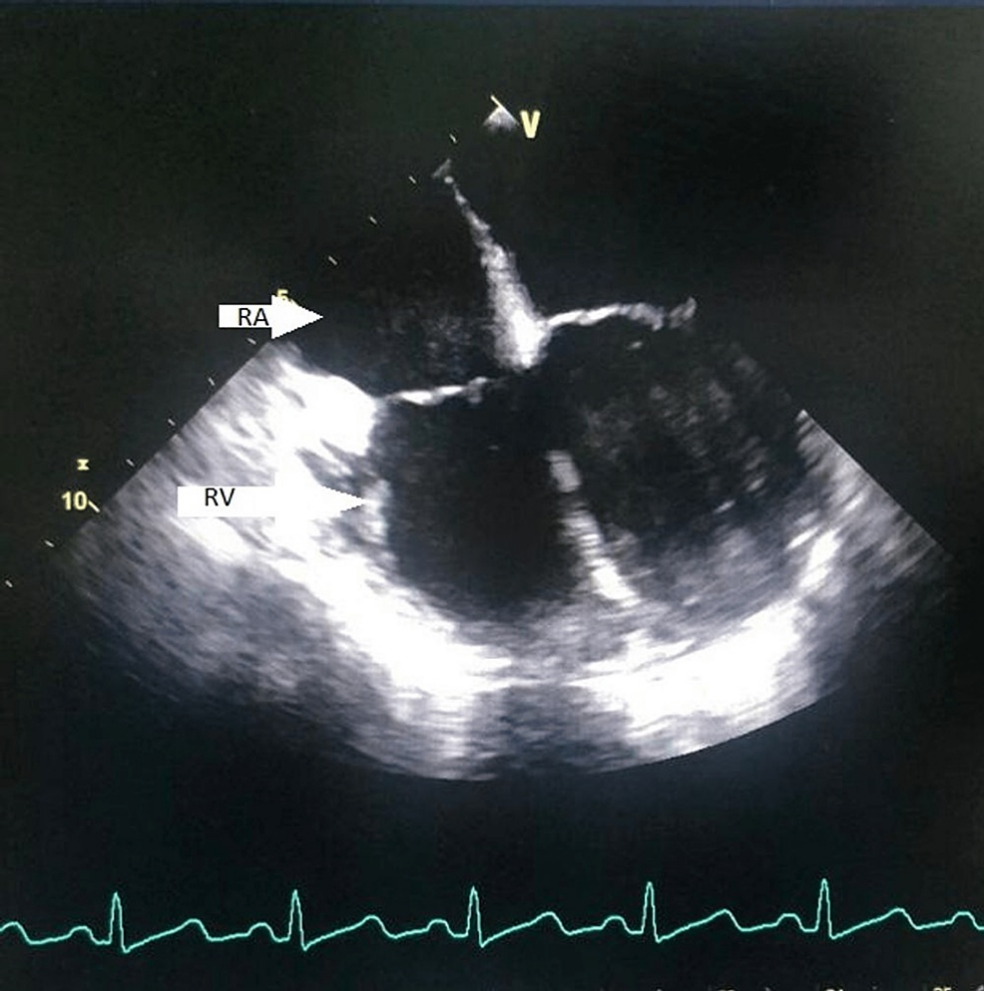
TEE showing mild RV/RA dilatation (arrows) TEE: transesophageal echocardiography; RV: right ventricle; RA: right atrium
